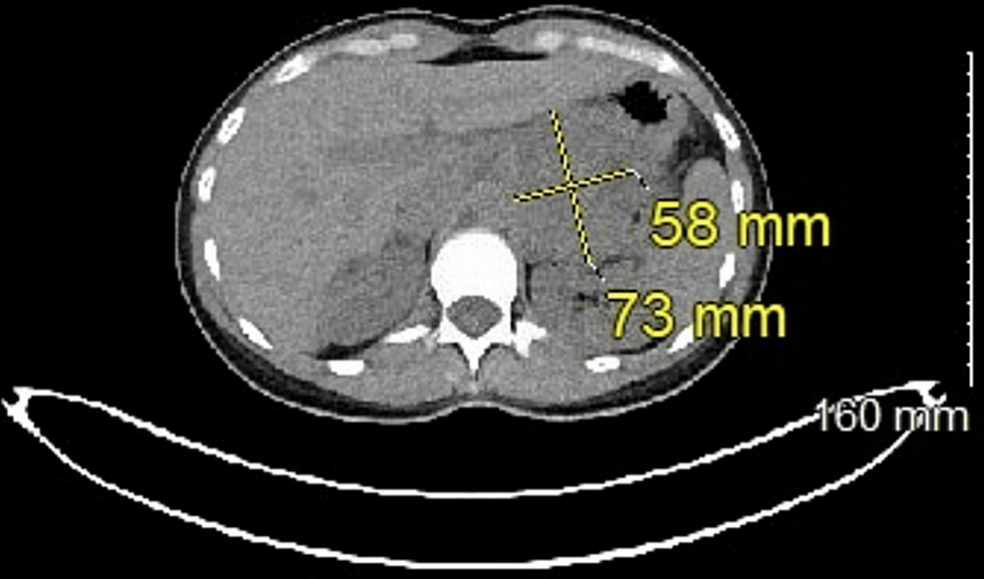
Computed tomography showing 7.3 x 5.8 cm left adrenal tumor
